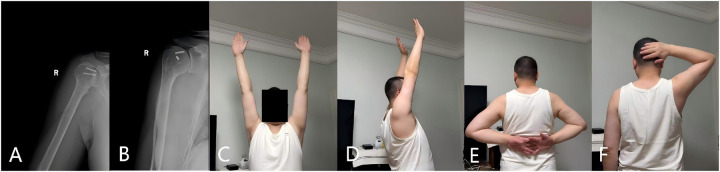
(A,B) Radiographs obtained 1 year after revision surgery show complete fracture union. The plate and several screws were removed; two screws were retained because they were located within the joint capsule. No radiographic signs of humeral head osteonecrosis are present. (C–F) At 1 year postoperatively, shoulder function had recovered well.
